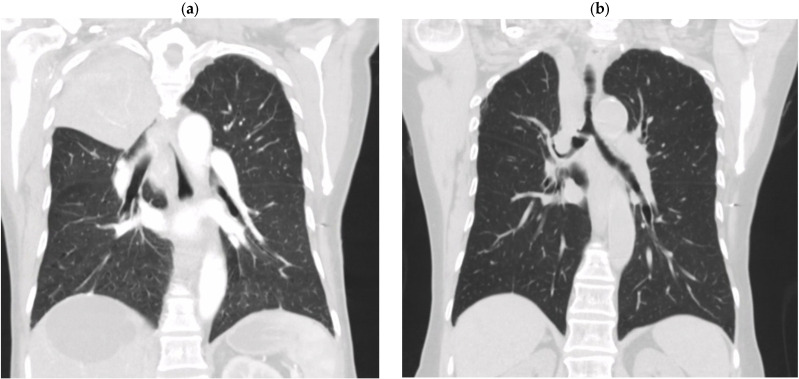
Evolution of chest CT imaging in Case 1: (a) before treatment—December 2021, (b) after treatment—December 2023. Interval improvement of dense consolidation in the right upper lobe.
